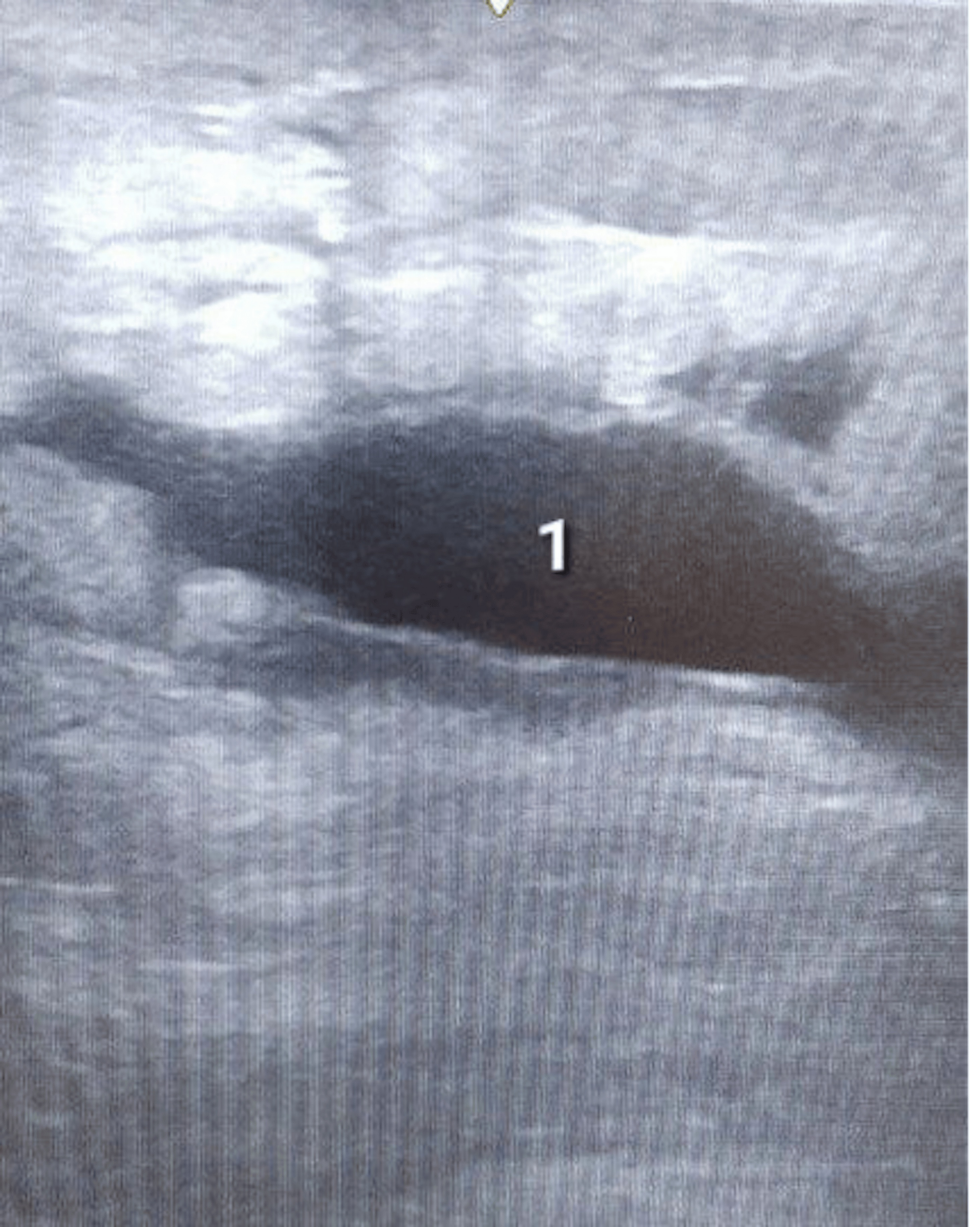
Abdominal wall ultrasound on postoperative day four showing hypoechoic fluid collection (exudate) in the subcutaneous adipose tissue.
